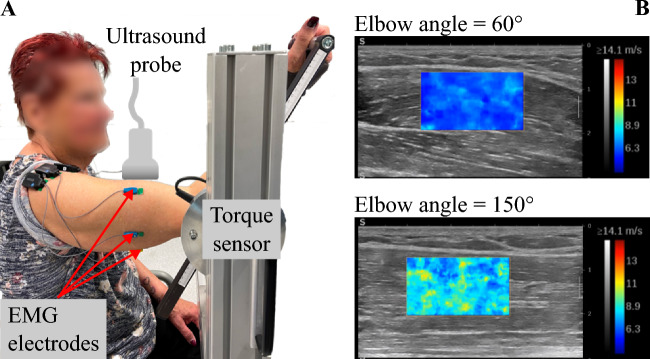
(A) Experimental setup. The participant’s elbow joint axis is aligned with the rotational axis of the torque sensor. Surface electromyography (sEMG) electrodes are positioned at the biceps brachii and triceps brachii muscles. The ultrasound probe is placed on top of the biceps brachii muscle belly and aligned with the muscle fiber direction. (B) Exemplary ultrasound frames including shear wave propagation speed color overlays during 50% isometric ramp contractions for two of the elbow angles (60°, 150°) studied.
